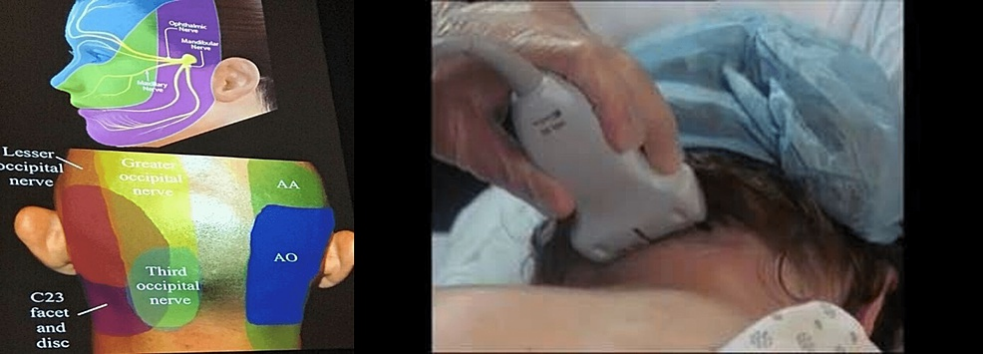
Treating pain at the third occipital nerve and C2-3 facet and disc using ultrasound guidance. In the top left image, the main nerves associated with face pain are indicated as they traverse the face. In the bottom left image, the nerves and C2-3 facet are shown from a posterior view. Note the lesser occipital nerve at the top and the third occipital never below. The right-hand image shows the ultrasound technician obtaining the images of these nerves and the facet and disc. AA, atlantoaxial; AO, atlanto-occipital
